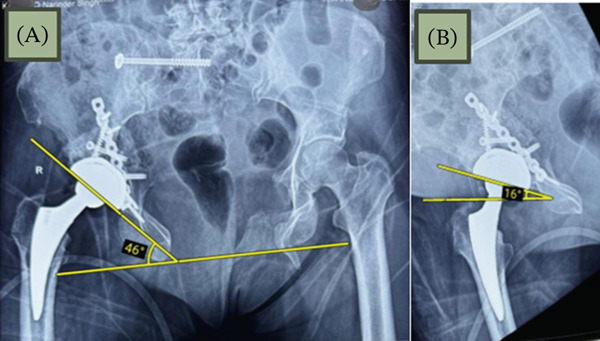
(A, B) Immediate postoperative anteroposterior pelvic radiographs demonstrating satisfactory acetabular component positioning with an inclination of 46° and anteversion of 16°.
